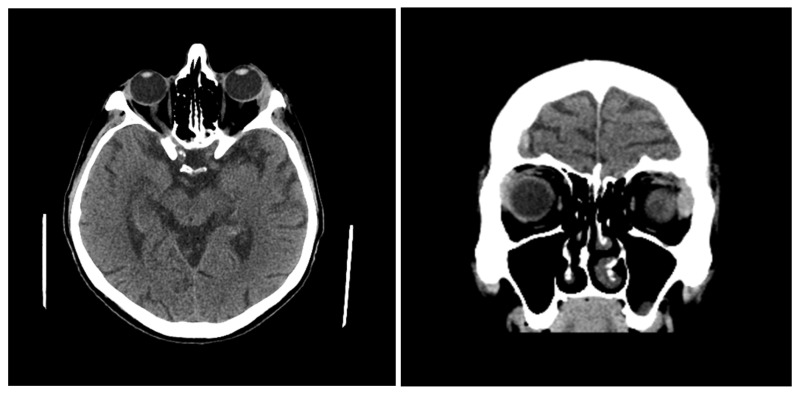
Axial (left) and coronal (right) CT scans of the endocranium showing enlargement of the left lacrimal gland (17 × 7.7 mm).
